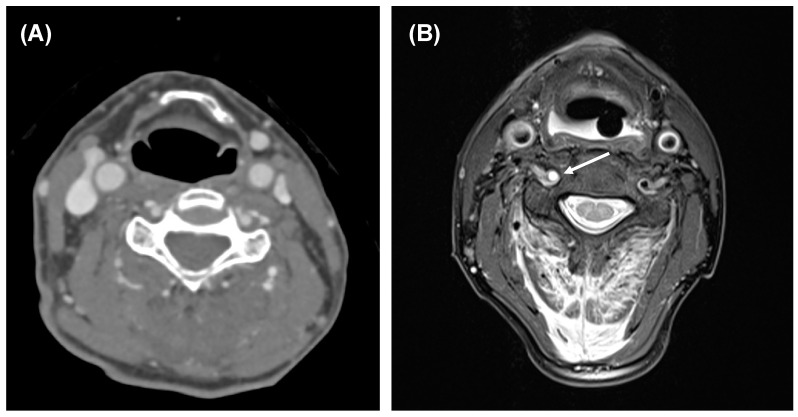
CT and MRI of a 67-year-old male after a car accident. (A) CT including CT-A revealed a fracture of C2. (B) Additional MRI showed a dissection of the right vertebral artery (arrow; proton density image with fat-saturation), progressed in the meantime from a smaller injury not detectable in CT-A. Furthermore, MRI showed additional fractures of C4 and C5 and a myelon damage level C2.
